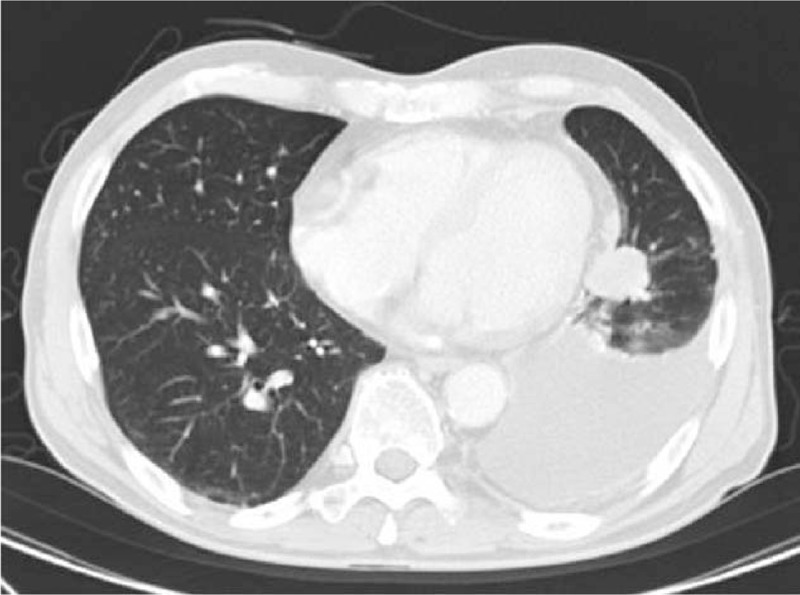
Chest computed tomography (CT) in August 2007. Chest CT scan showing a nodule in the left lower lobe with left pleural effusion.
